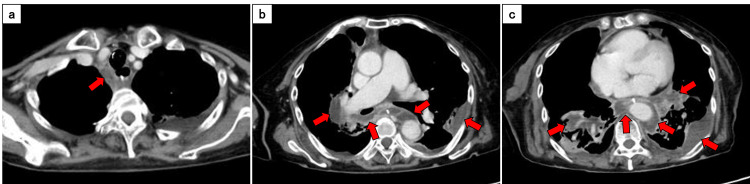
Contrast-enhanced computed tomography on postoperative day 8 in the axial plane (a-c) Slight progression of low-density areas (red arrows) suggestive of abscess formation around the mediastinum, hilar region, thoracic aorta, and right hypopharyngeal region. Note the mild increase in the left pleural effusion.
